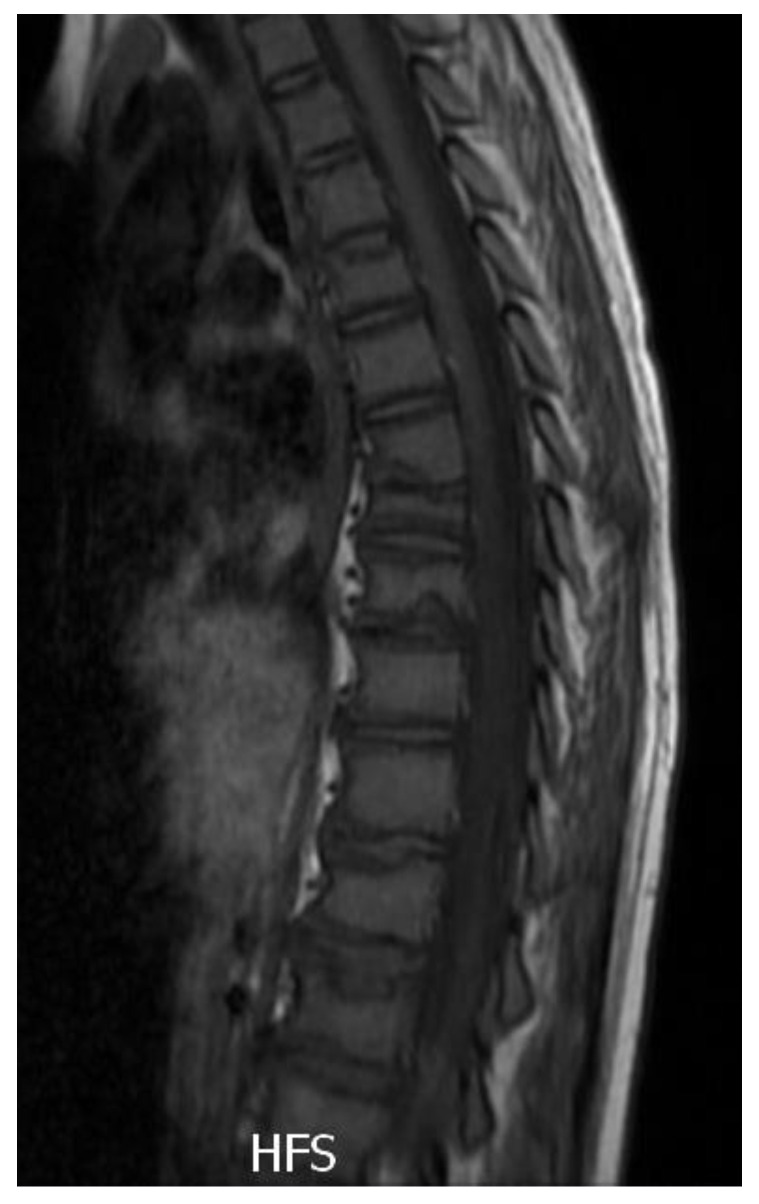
MRI of the spine after 1 year of pamidronate therapy (4th pamidronate cycle).
